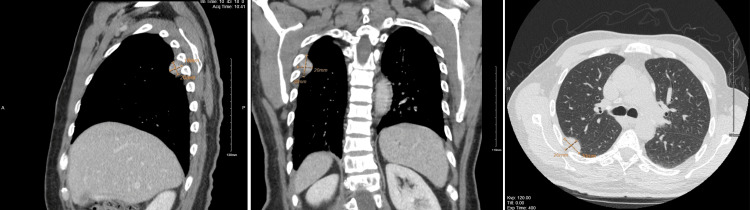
Chest CT findings of the right pleural mass, measuring 30 x 20 mm in maximum dimensions. They are shown in sagittal, coronal, and axial views. CT, computed tomography
